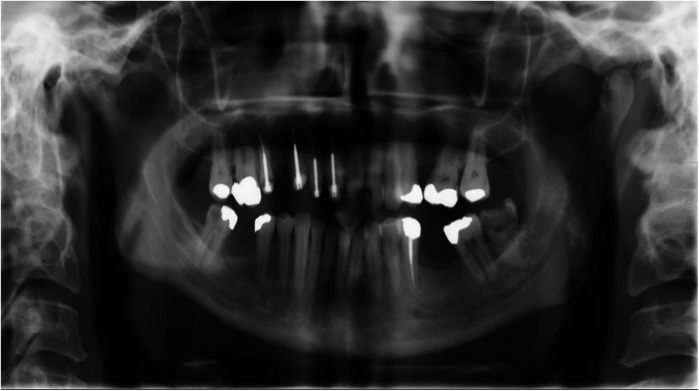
Panoramic radiogram taken upon admission to the emergency department. The left condyle appeares more pronounced compared to the right, showing an enlarged intra-articular space.
